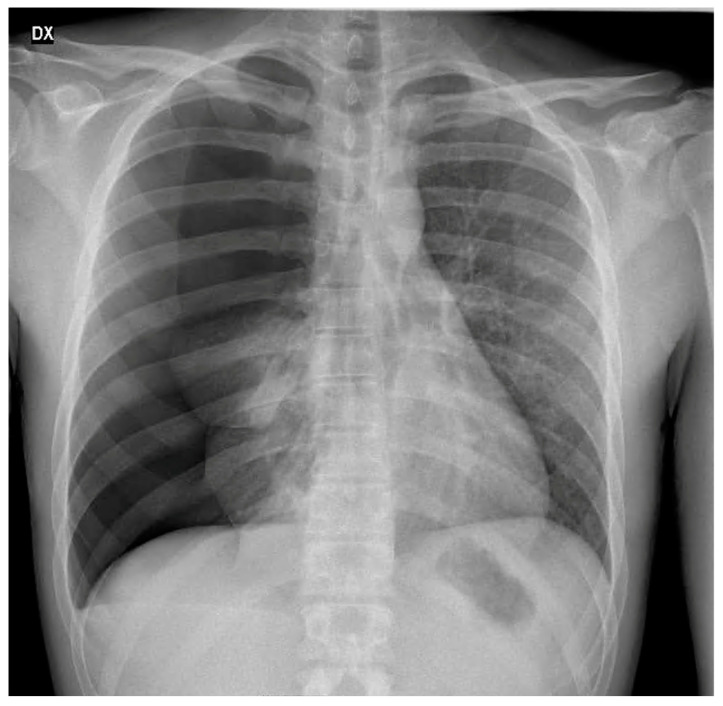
X-ray showing massive right idiopathic pneumothorax.
